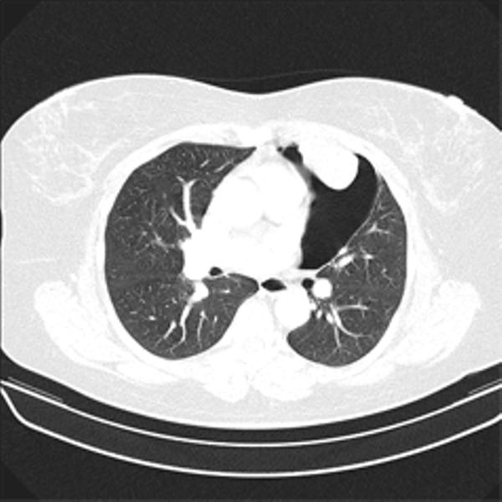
Chest computed tomography showed an 3 × 5 cm measuring intrathoracic tumor with broadly based contact to the pericardium, surrounded by pneumatocele. It was identified as an extrapulmonary, mediastinal benign solitary fibrous tumor.
